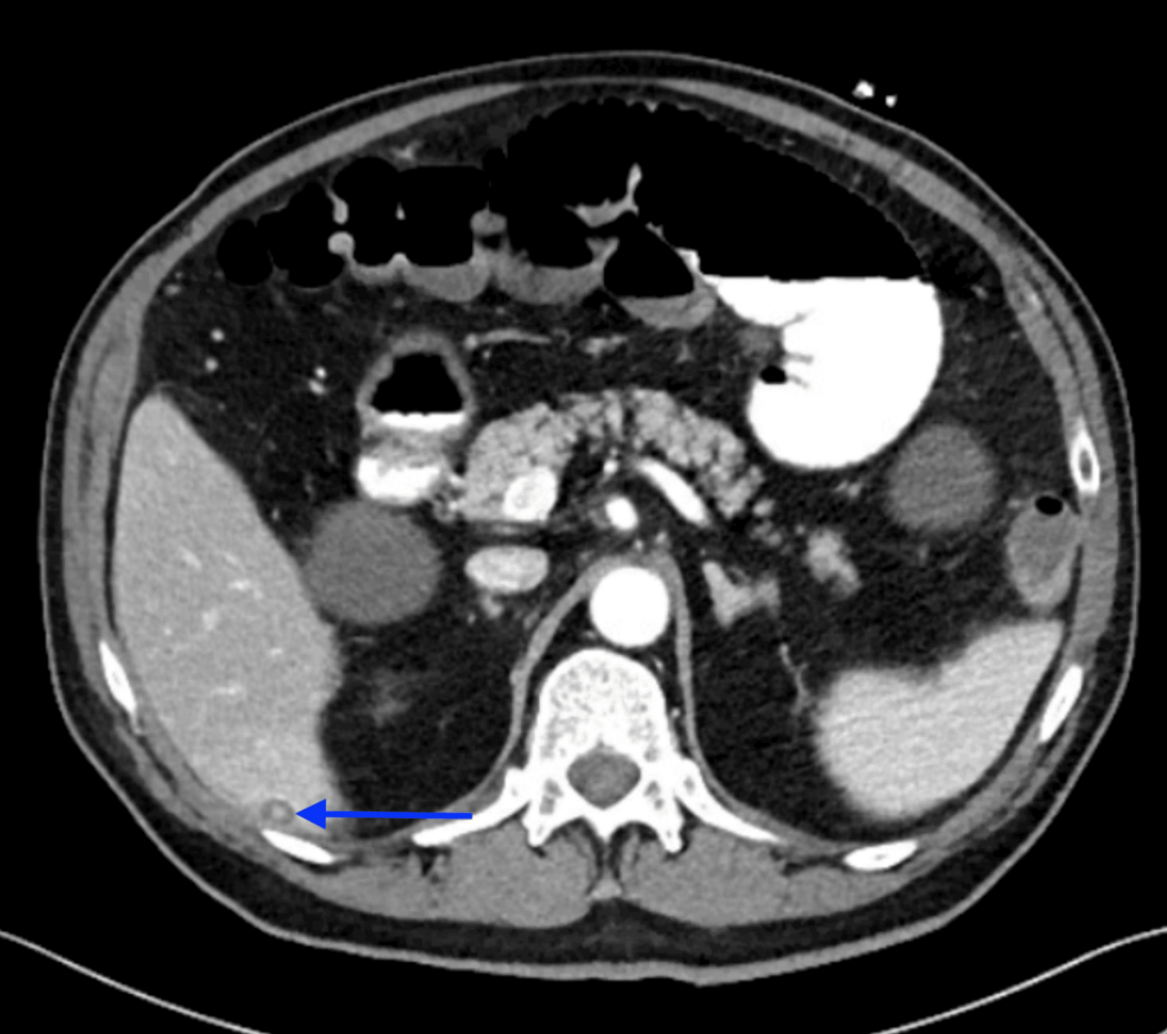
Post-operative CT scan obtained several weeks after appendectomy revealing a small round radiodensity along the posterior surface of the liver within the subhepatic space, retrospectively consistent with a retained appendicolith that later served as the nidus for abscess formation.
